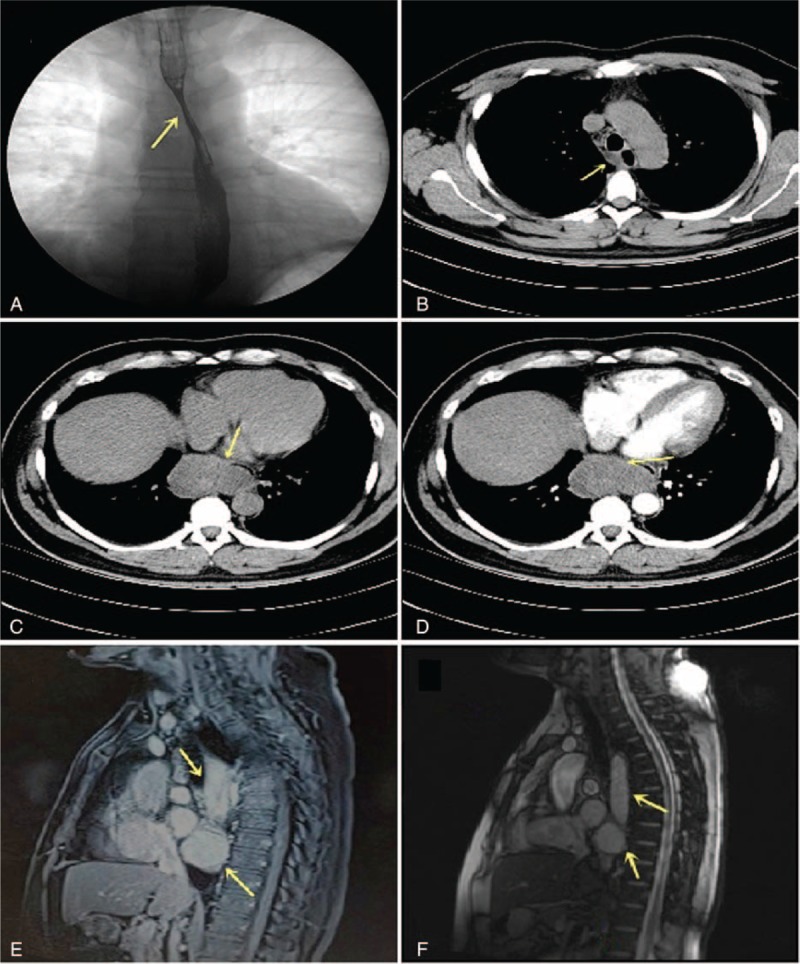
Preoperative imaging diagnoses. (A) A barium swallow identified an extrinsic compression shadow ∼13 cm in length under the aortic arch (arrowheads), with a regular mucosal pattern. (B) The esophageal computer tomography revealed a sharply defined mass attached to the right side of esophageal wall. (C) The maximum cross-section area was 7.2 × 4.3 cm and presented dumbbell-shaped (arrowheads). (D) Parenchyma was heterogeneous with slight enhancement on contrast material-enhanced CT scan. (E,F) Magnetic resonance imaging (MRI) images show a large circumscribed (a maximum cross-section area was 4.5 × 7.3 cm) tubular cystic lesion and a round mass behind the trachea, concomitant with compressed esophagus. The masses had a relatively high-intensity on both T1- and T2-weighted images. CT = computerized tomography, MRI = magnetic resonance imaging.
